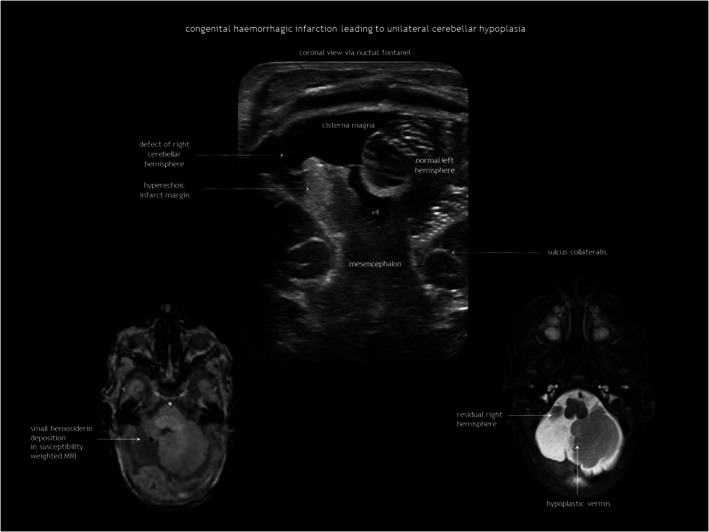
Unilateral cerebellar hypoplasia. Infant born preterm at 31 weeks of gestation with antenatal haemorrhagic disruption of the cerebellum leading to severe hypoplasia of the right cerebellar hemisphere and inferior vermis. Abbreviation: MRI, magnetic resonance imaging.
